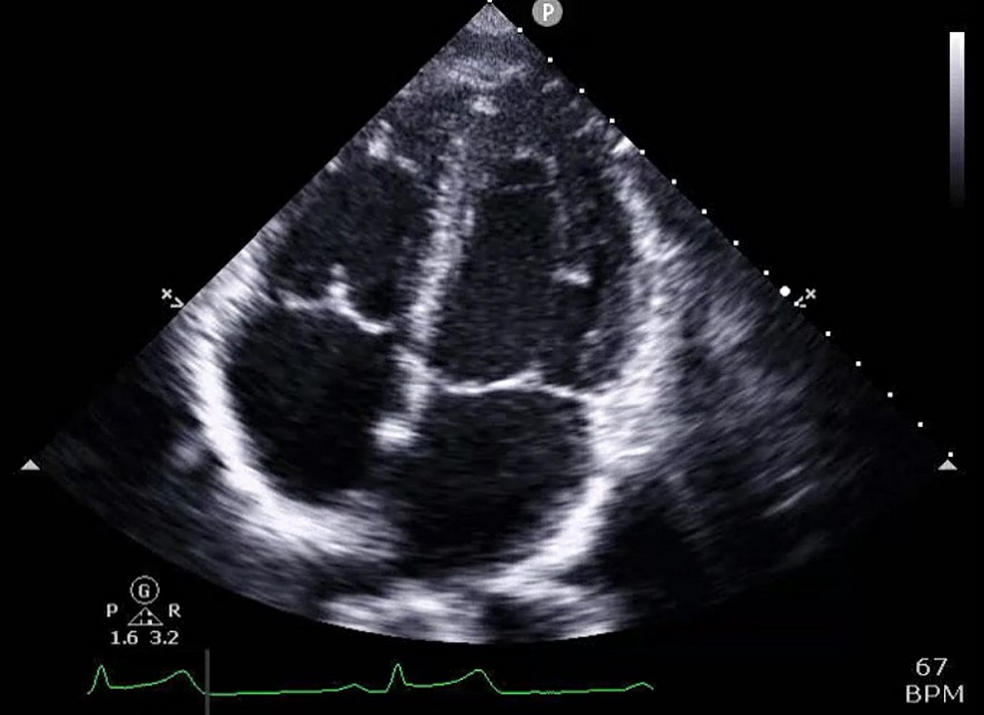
Apical view of the heart on trans thoracic echocardiogram
